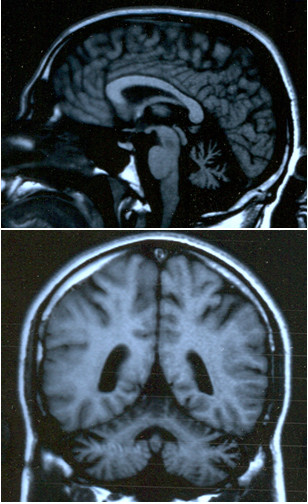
Brain MRI images of the younger patient (II-14) at age 25 years old, showing cerebellar atrophy
